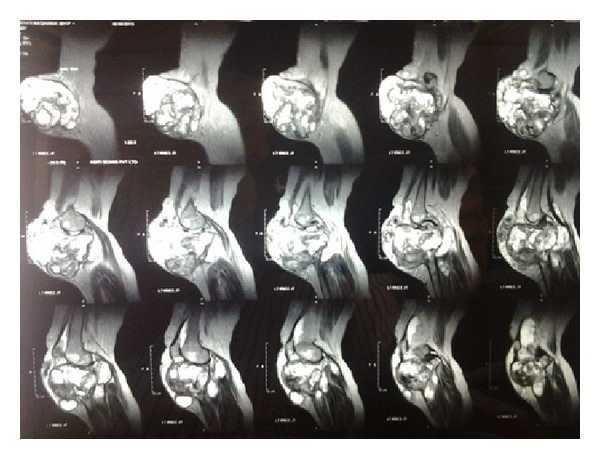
MRI of the right knee joint.
